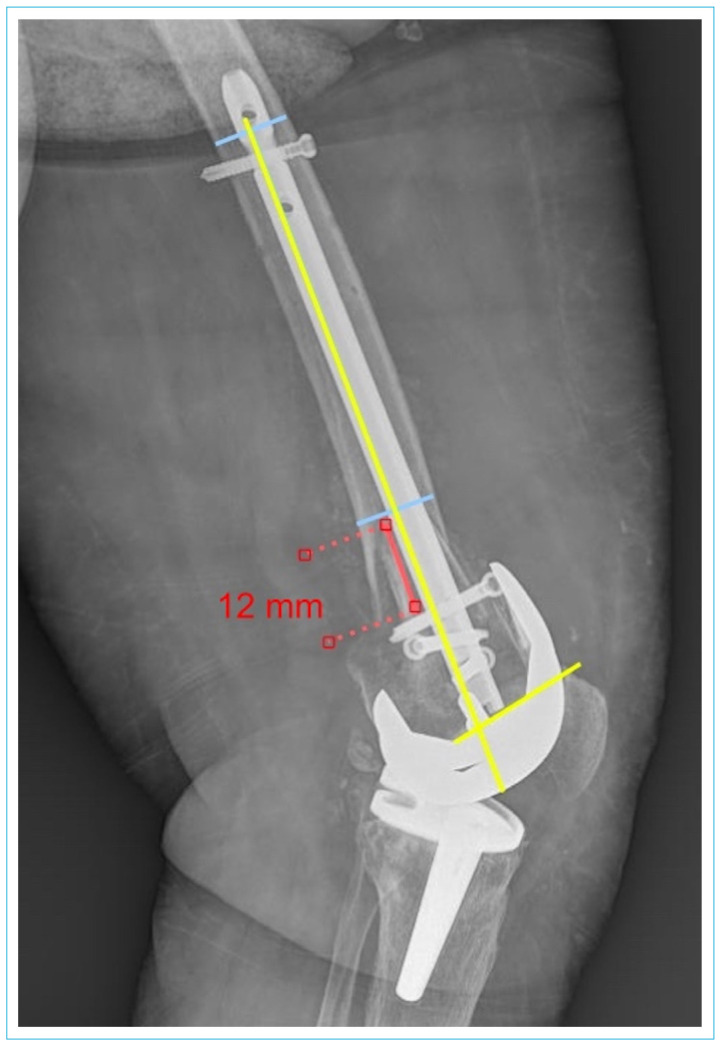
Lateral X-ray of the patient. The shortening was 12 mm, gamma angle: 80°.
